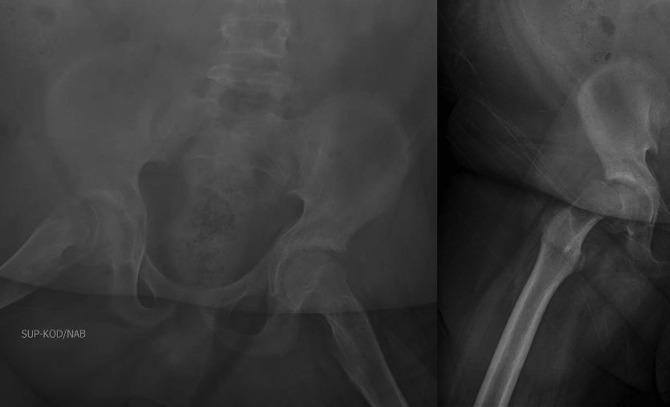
Radiographs at time of presentation—AP pelvis and lateral view of the right hip.
